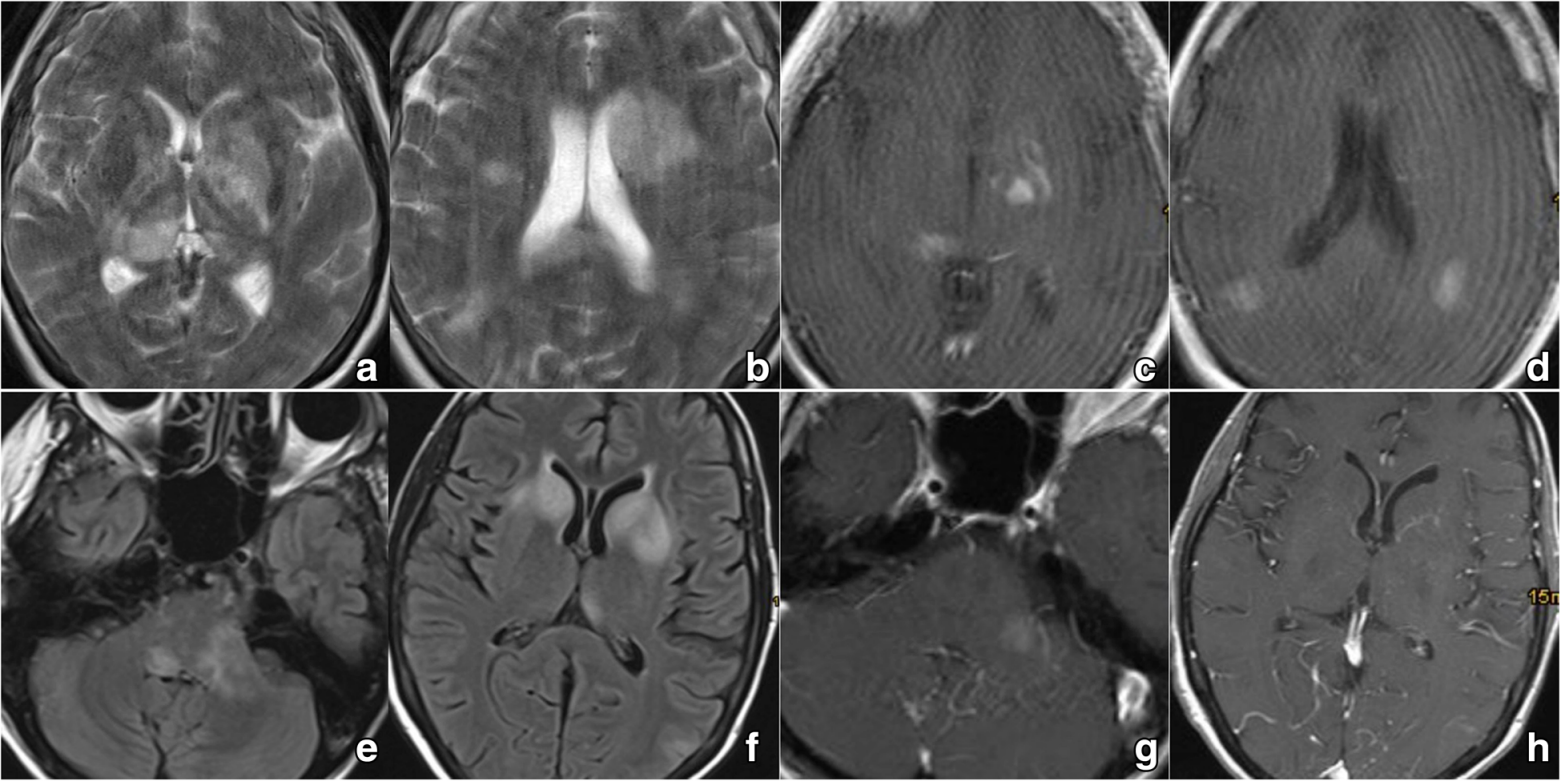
Cranial imaging of # 1, 2: a, b/e, f) MRI T2 flair showing multifocal hyperintense lesions with contact to the CSF space in both patients; c, d/g, h) MRI T1 with contrast showing only sparse enhancement in both patients
